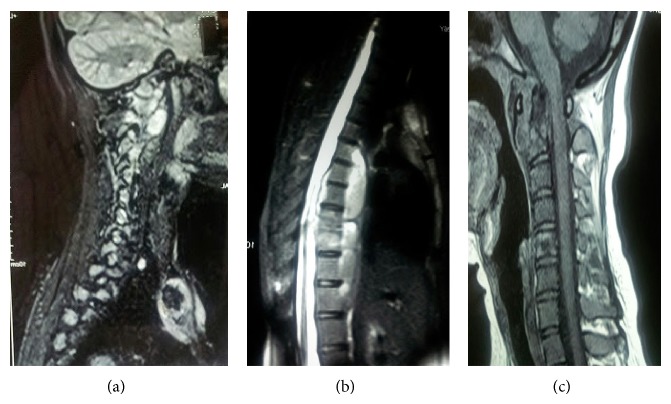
MRI images showing involvement of sternoclavicular joint along with dorsal and upper cervical involvement.
